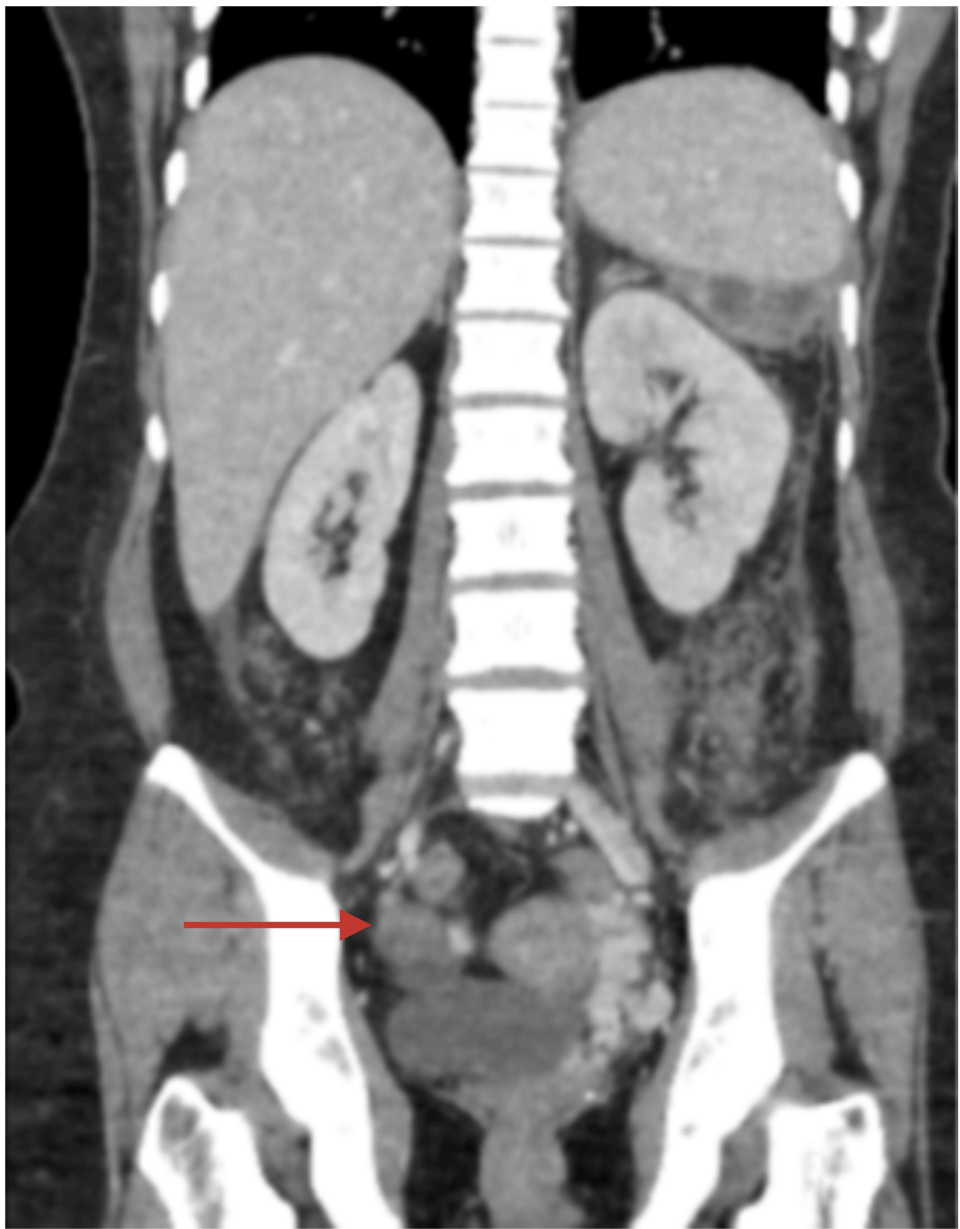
Coronal CT of the abdomen and pelvis showing right ovarian mass (red arrow) CT: computed tomography
